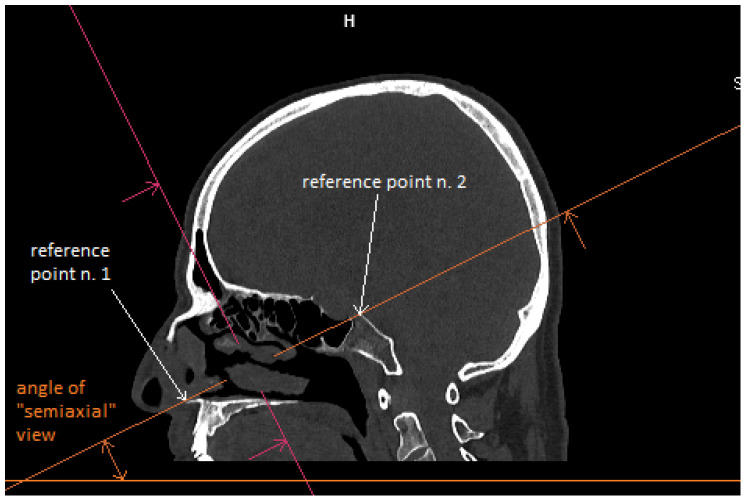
CT scan, sagittal view, creation of semiaxial plane (orange line), reference point n. 1–spina nasalis anterior; reference point n. 2–dorsum sellae.
